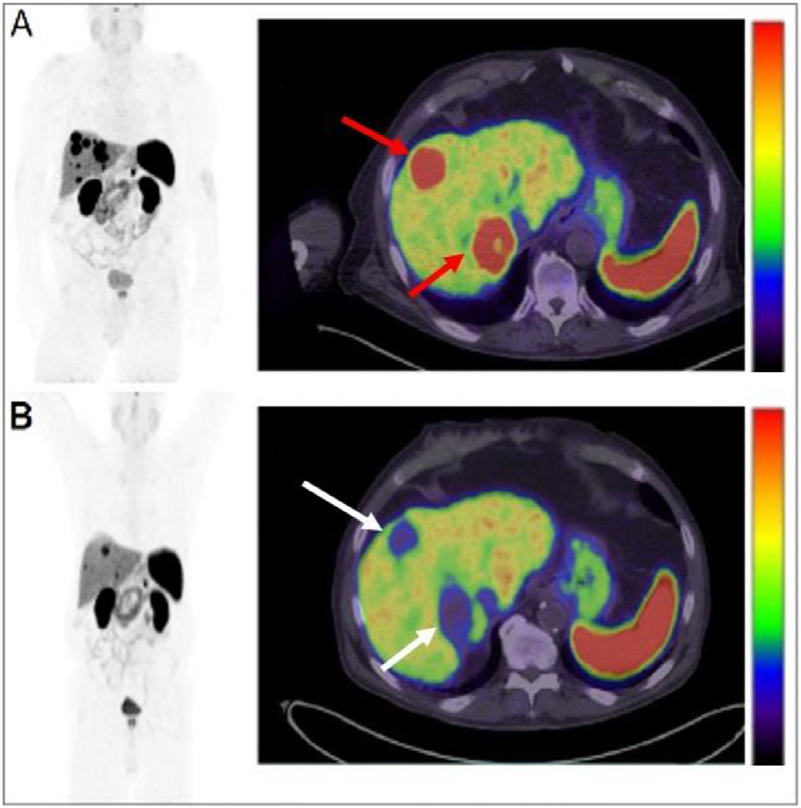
Whole-body Maximum intensity projection (MIP) image and axial fused DOTATATE PET/CT image at the level of the liver and spleen, windowed 0-15 SUV prior to radioembolization (A) demonstrate multiple DOTATATE avid hepatic lesions (red arrows on axial fused image; also evident on MIP image). The segment 4 and 7 lesions were mapped, selectively catheterized, and treated with Y90 TheraSpheres at a dose of 300 Gy. Following radioembolization, MIP and axial DOTATATE PET/CT (B) show complete resolution of DOTATATE avidity in the previously identified hepatic metastases, consistent with complete response to radioembolization therapy (white arrows). Few smaller persistent hepatic metastases, as evident on the MIP image, were not targeted by Y90 therapy.
